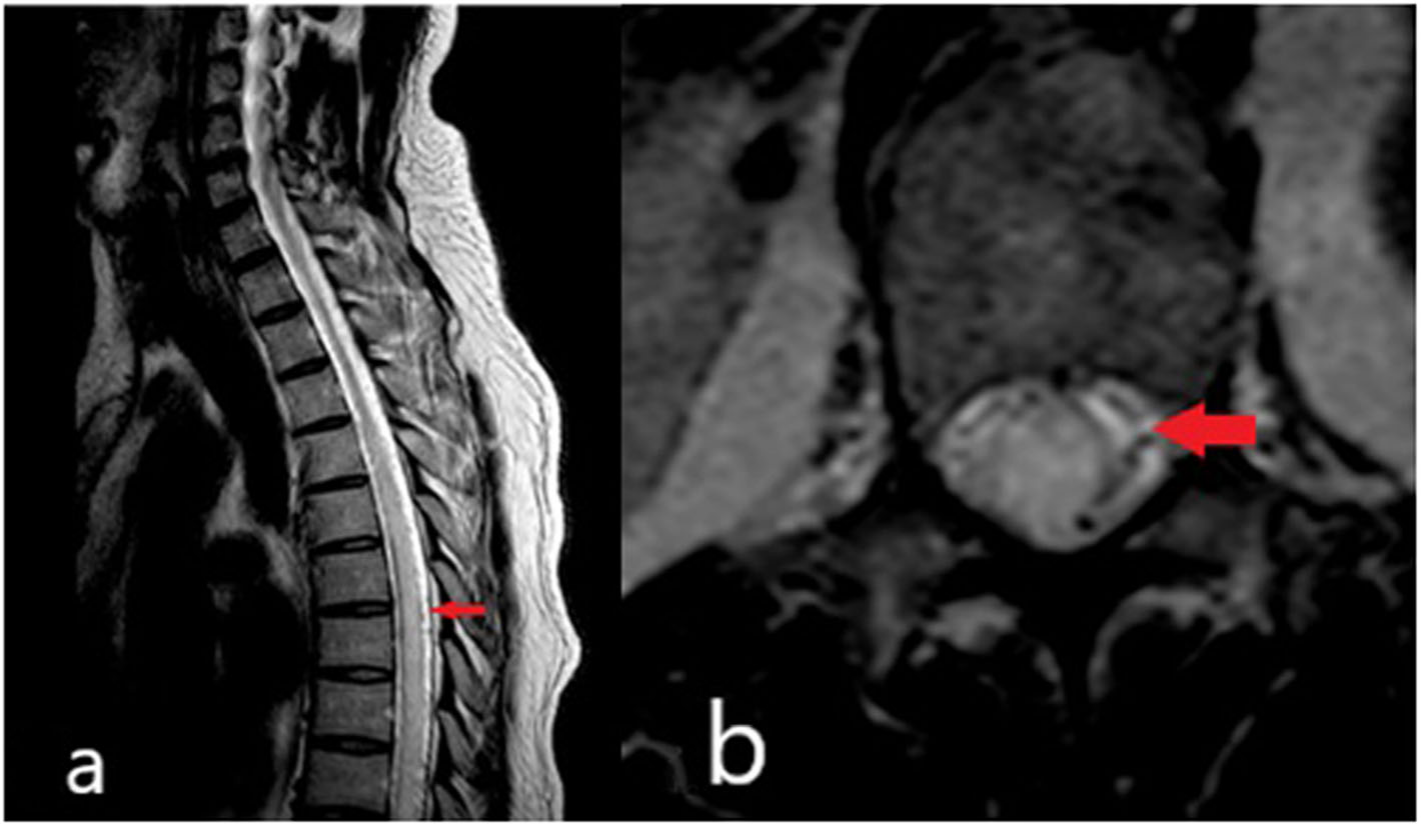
a and b MRI of patient 2, showing the suspicious dilated vessels at the posterior aspect of the thoracic cord (red arrow)
